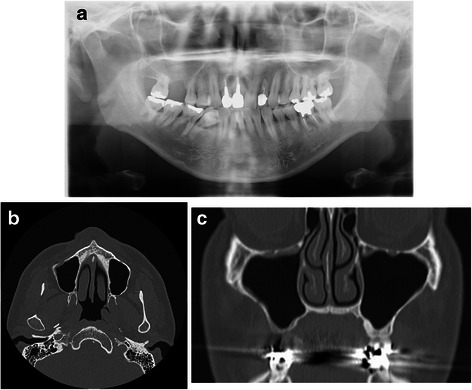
a Panoramic radiograph obtained 1 week after surgery. b, c Computed tomography images obtained 6 months after surgery (b, axial section; c, coronal section)
